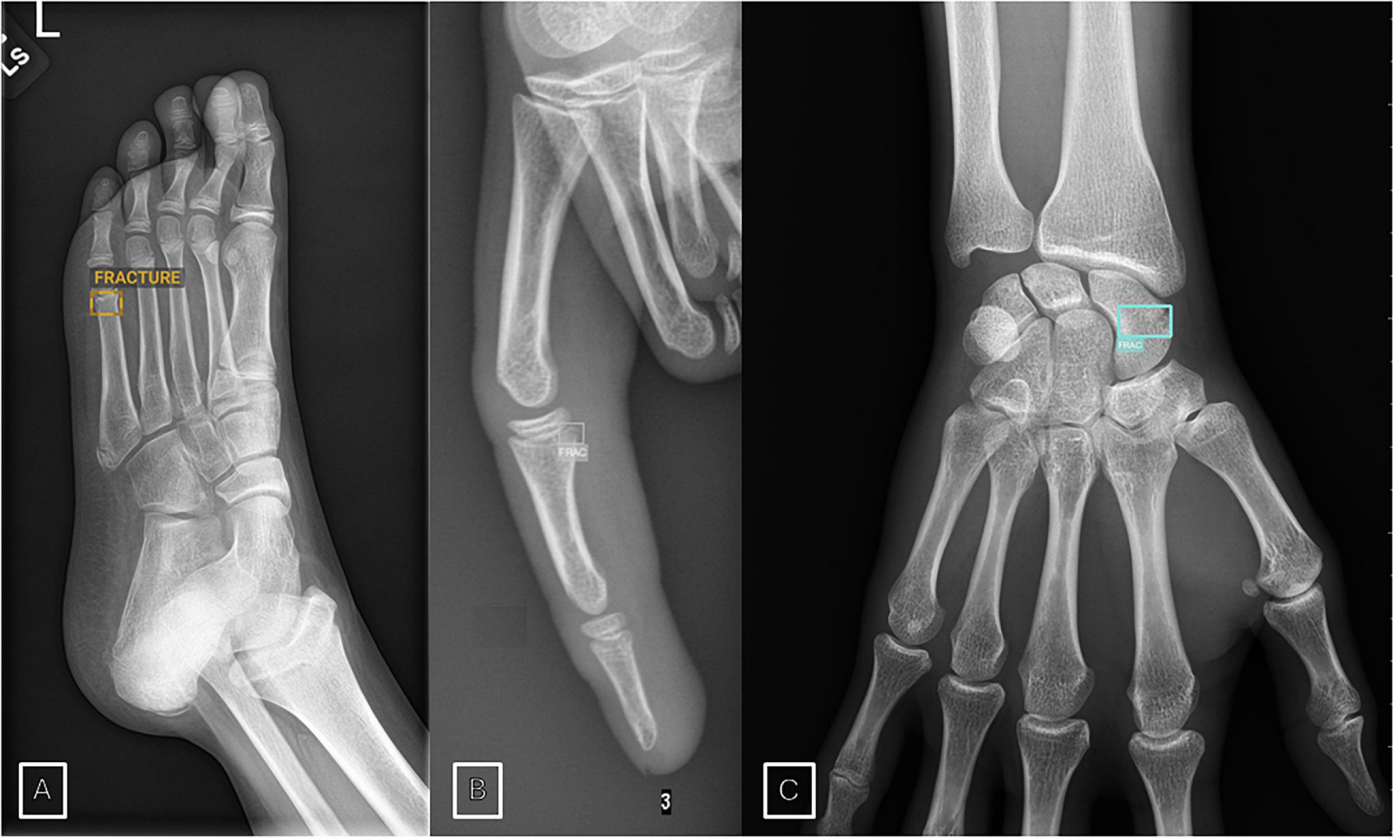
AI detection of subtle fractures. Distal metaphyseal buckle fracture of the fifth metatarsal bone detected on radiograph using AI software (Boneview by GLEAMER) (A). Phalangeal fracture detected by another AI tool (MILVUE Suite 2.4.1) (B). Scaphoid fracture detected using MILVUE Suite 2.4.1 (C).
